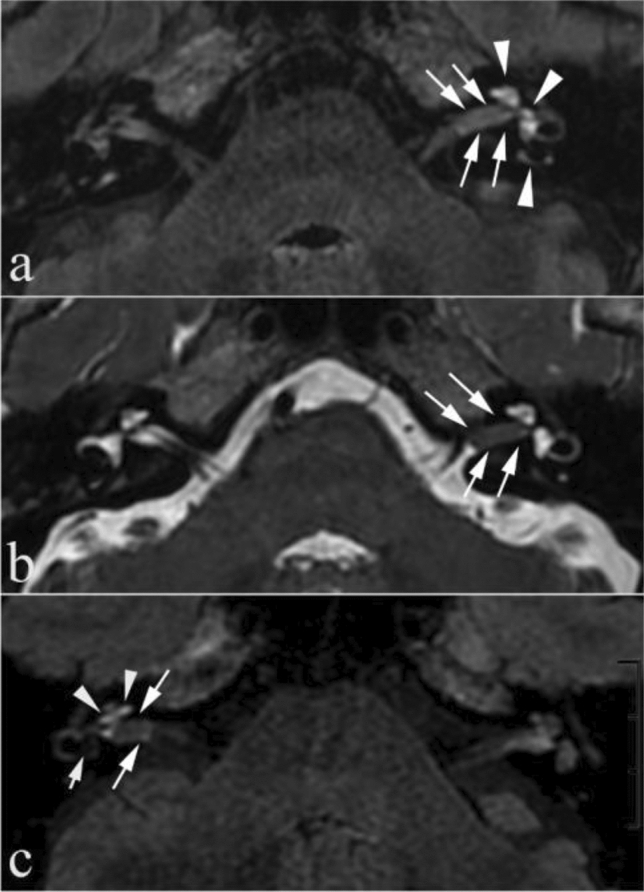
A 50-year-old woman with left vestibular schwannoma (a, b) and a 49-year-old man with right vestibular schwannoma (c) in the internal auditory canal. The inner auditory canal schwannoma presented relatively equal hyperintense (long arrows) on intravenous gadolinium-enhanced 3D-FLAIR (a, c) and asymmetric hypointense (long arrows) on 3D-T2WI (b) compared with the contralateral side, and the nerves in the internal auditory canal were unclear. Axial 3D-FLAIR image (c) showed vestibular hydrops grade III (short arrow). Note: abnormal cochlear (arrowhead) and/or vestibular (arrowhead) perilymphatic enhancement was shown on the same side compared with the contralateral side (a, c).
